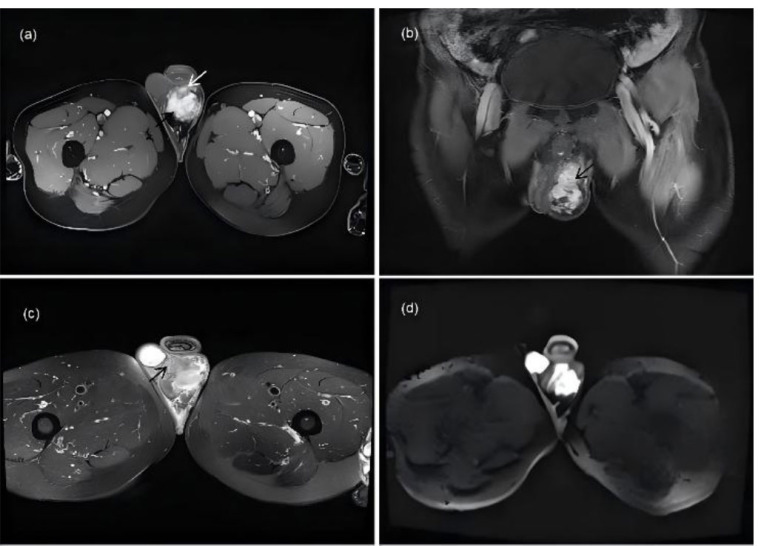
Pelvic Enhanced MRI. Axial and coronal contrast-enhanced T1-weighted images demonstrate a markedly enhancing lesion in the left epididymal region, indicated by black arrows (a and b). In the coronal view, the spermatic cord indicated by the white arrow demonstrates compression with ill-defined margins and enhancement (a). Axial fat-suppressed T2-weighted image shows a lesion with an isointense signal accompanied by an internal heterogeneous signal (c). Diffusion-weighted imaging (DWI) sequence exhibits significant restricted diffusion within the lesion (d).
